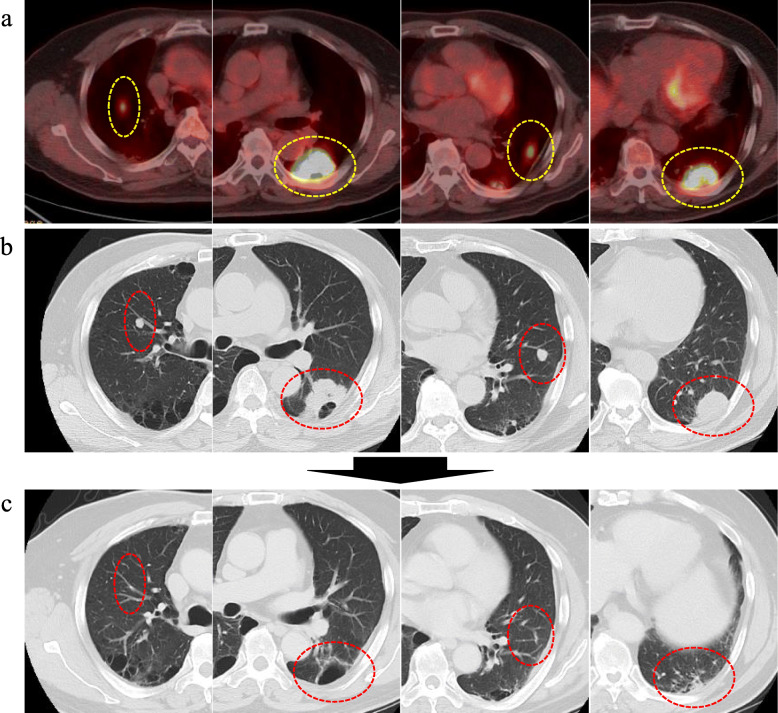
Preoperative images of the lung tumor. a Positron emission tomography (PET) before pembrolizumab therapy shows multiple tumors in bilateral lobes of the lungs (dotted circles). Marked fluorodeoxyglucose (FDG) uptake is observed in each panel (standardized uptake value (SUV) max 14.7 in the second left panel, for example). b Chest computed tomography (CT) before pembrolizumab therapy shows 4 nodules corresponding to each point of PET-CT (dotted circles). c CT imaging after pembrolizumab therapy. Almost complete remission is seen (dotted circles)
